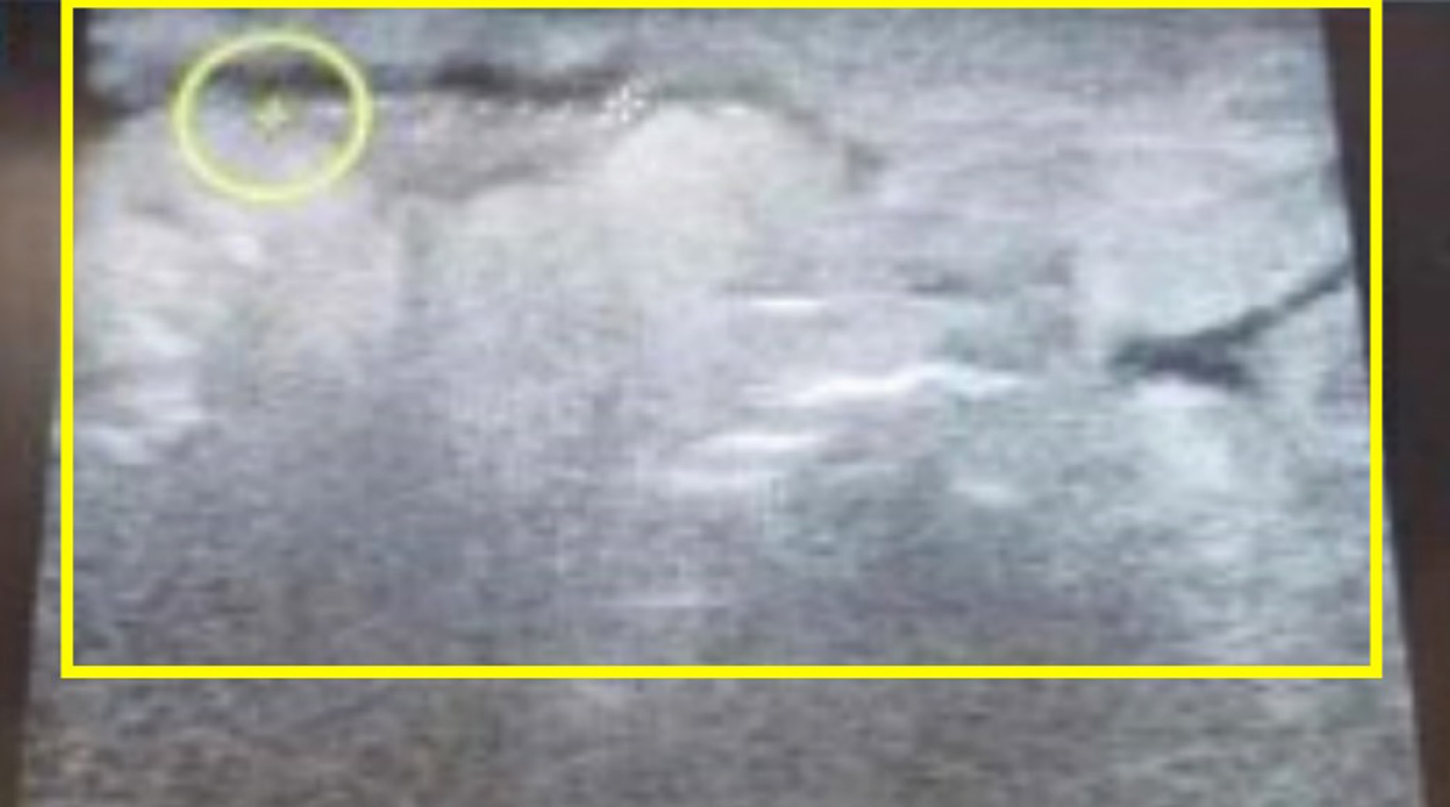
Imaging obtained from a bedside ultrasound machine in the Emergency Department. The yellow box displays an area of induration at the inferior portion of the right breast as well as soft tissue edema with no discrete drainable fluid collection.
